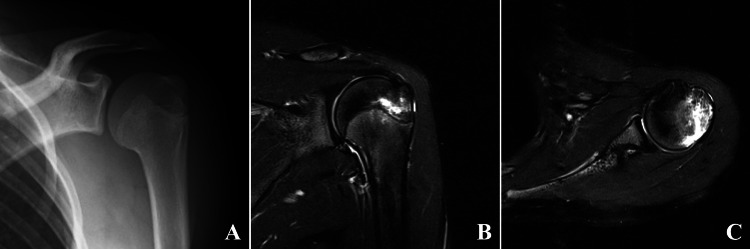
X-ray and MRI of the left shoulder at the first visit. A, Frontal view of the X-ray. B, Coronary MRI STIR slice. C, Axial MRI STIR slice. MRI, magnetic resonance imaging; STIR, short-tau inversion recovery.
